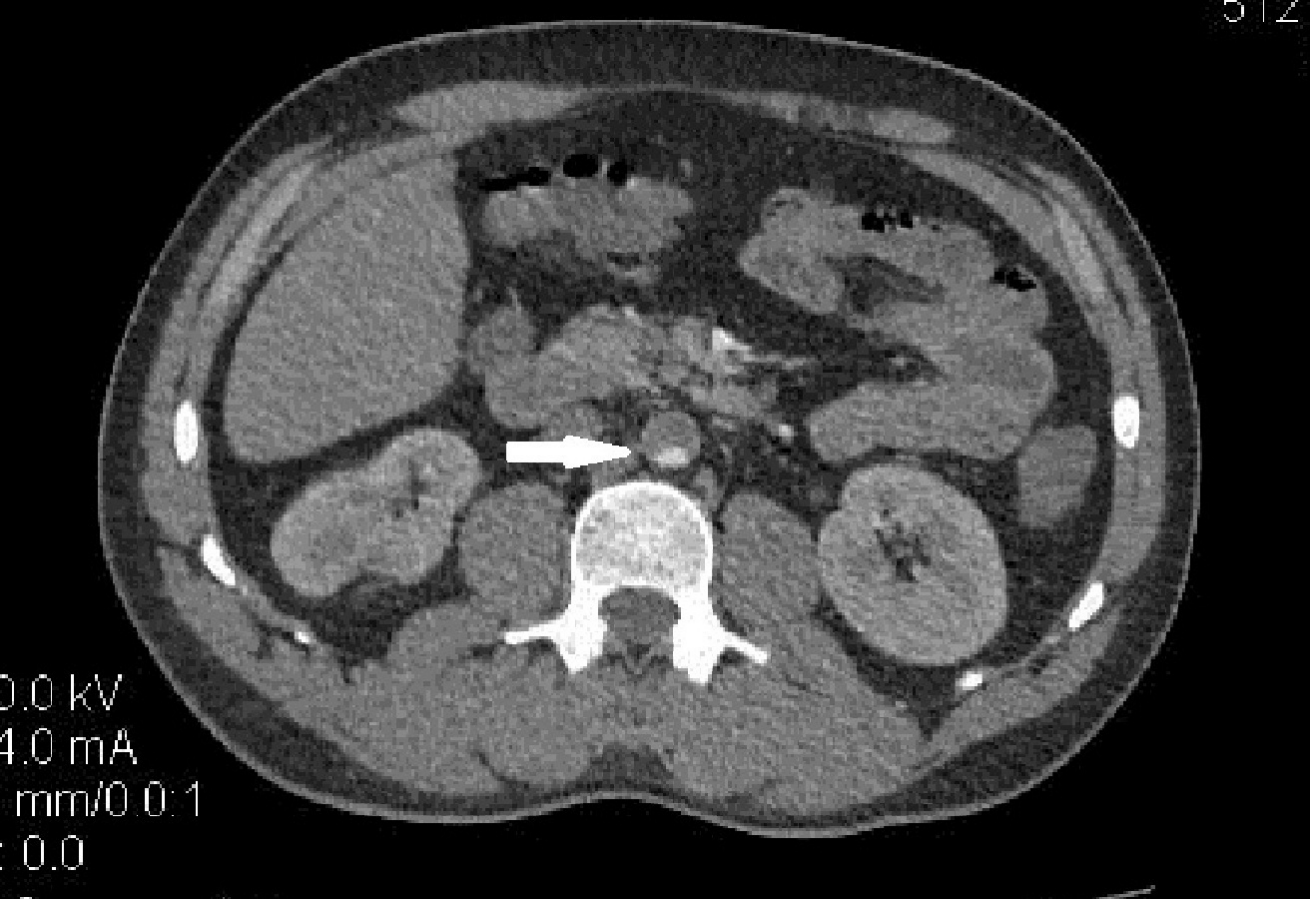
Computed tomography angiogram (CTA) of the abdomen. CTA shows a thrombosed abdominal aorta beyond origin of the renal arteries as indicated by the white arrowhead.
